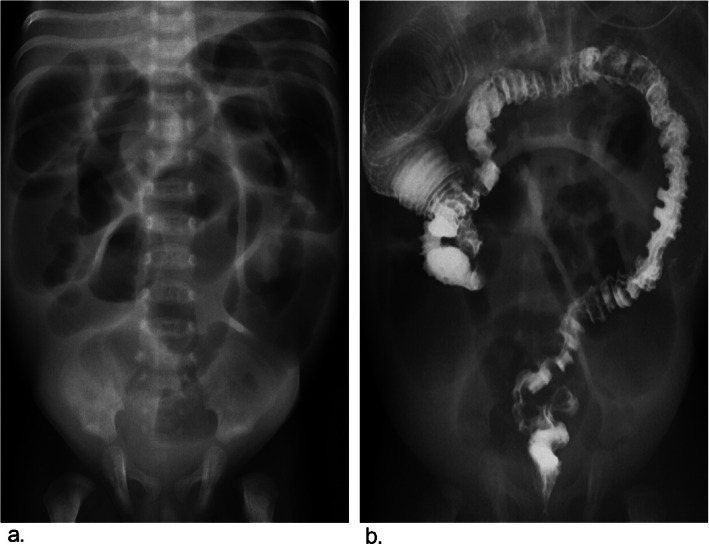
A patient with TCA has all common and typical radiographic signs of preoperative BEs: rectosigmoid index ≤ 1, transition zone, irregular contraction, gas-filled small bowel, a microcolon, question-mark-shape colon and ileocecal valve reflux
